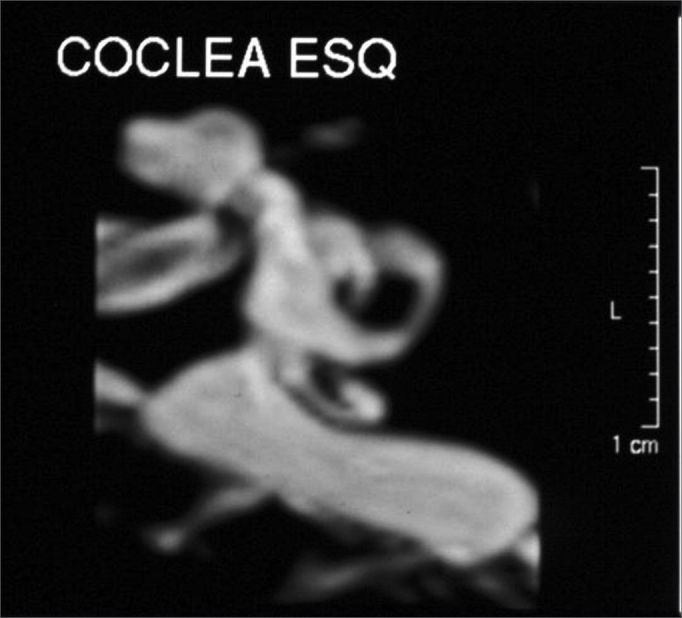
Bilaterally enlarged vestibular aqueduct - temporal bone MRI - axial view - T2 weighed submillimeter slice.
